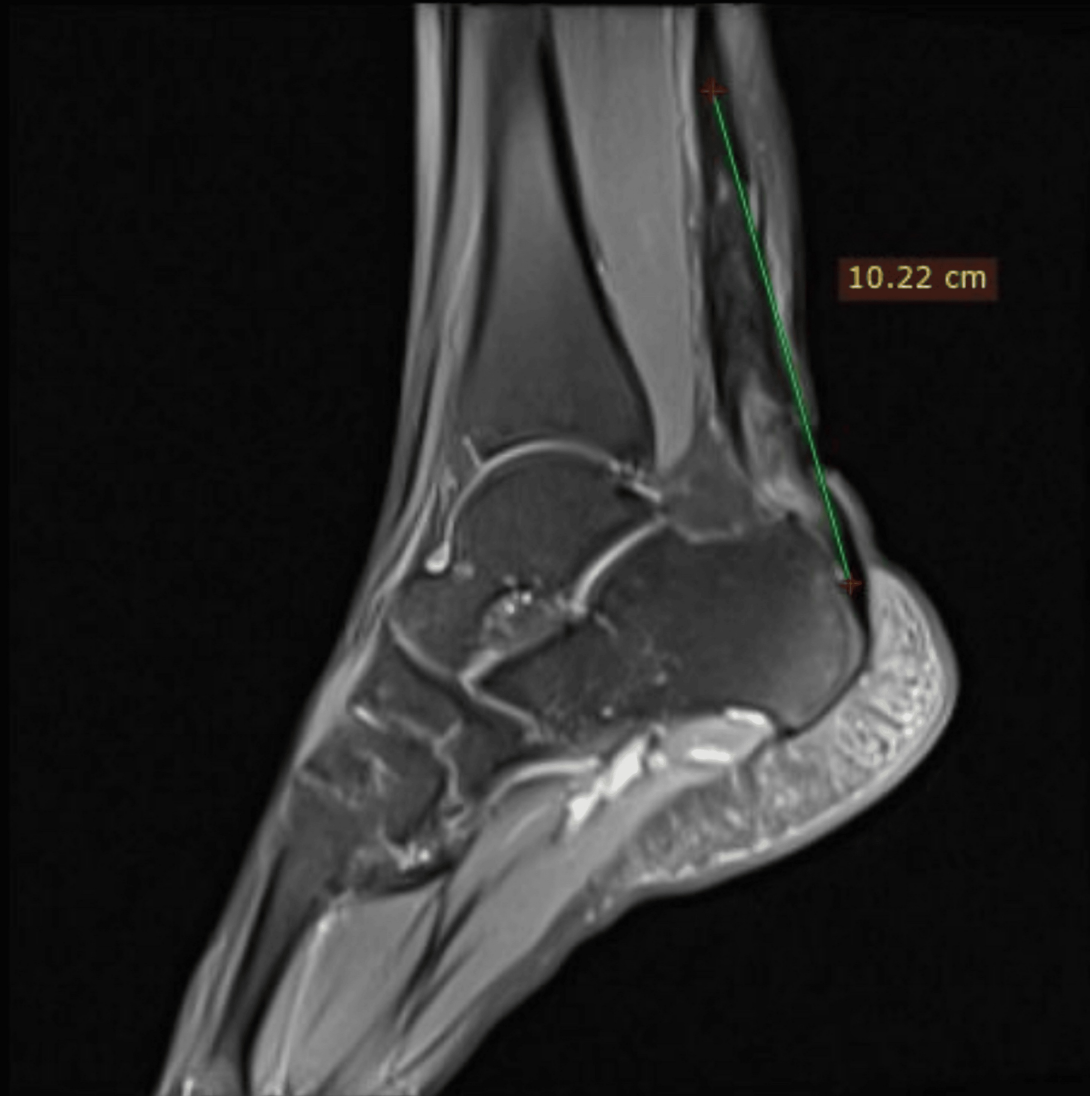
Pre-operative MRI of the ankle showing torn and retracted tendo-Achilles with gap of 10.22 cm Case 1
